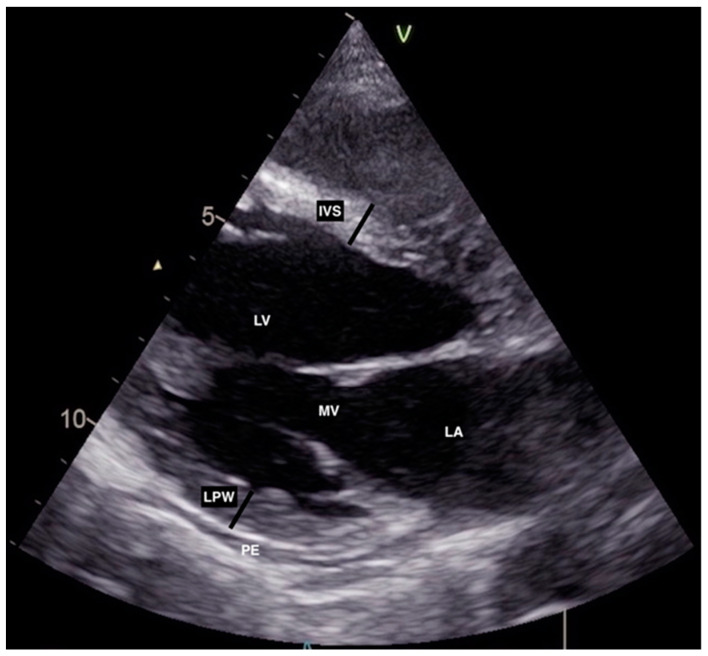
Initial transthoracic echocardiography showing left ventricular wall thickness (IVS, LPW) within normal range in parasternal long axis (PLAX) view. IVS: interventricular septum, LA: left atrium, LV: left ventricle, LPW: left posterior wall, MV: mitral valve, PE: pericardial effusion.
